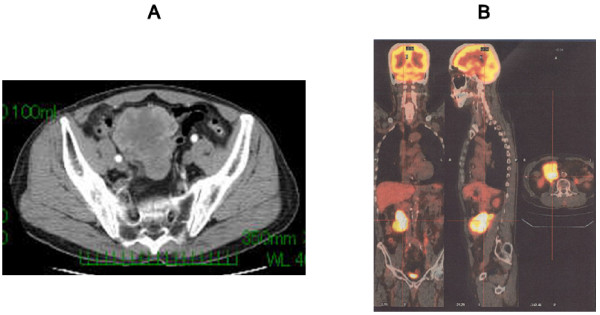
Preoperative imaging findings. A) CT scan shows a large, well-circumscribed, lobulated mass in the pelvis. The central region of the mass appears to have low density, while the marginal region is well enhanced. B) FDG-PET/CT shows a mass with increased FDG accumulation in the right lower abdomen, without any evidence of distant metastasis.
